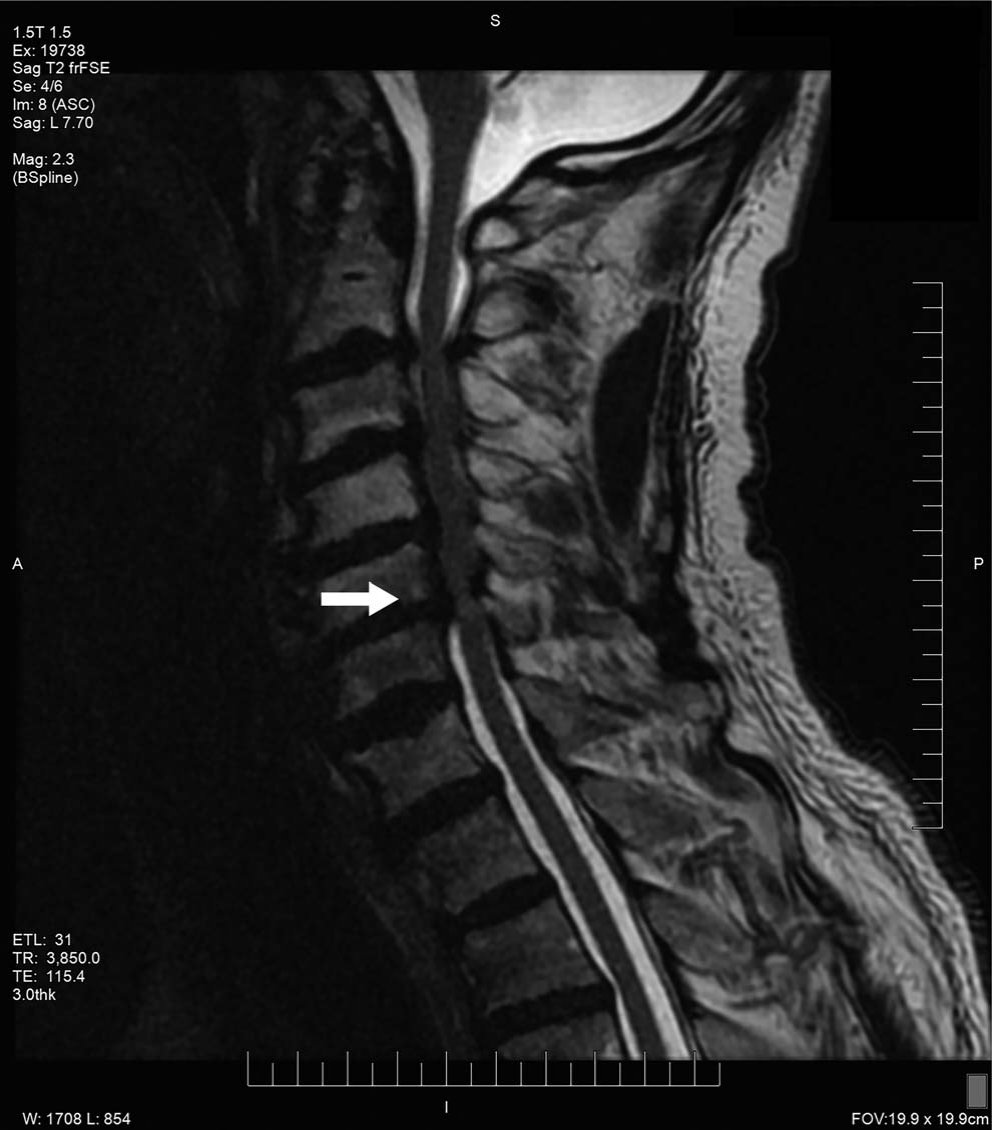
Preoperative T2-weighted magnetic resonance sagittal image of 63-year-old-male with severe cervical spinal stenosis demonstrates myelopathy with associated hyperintensity at the C5-C6 level (arrow).
